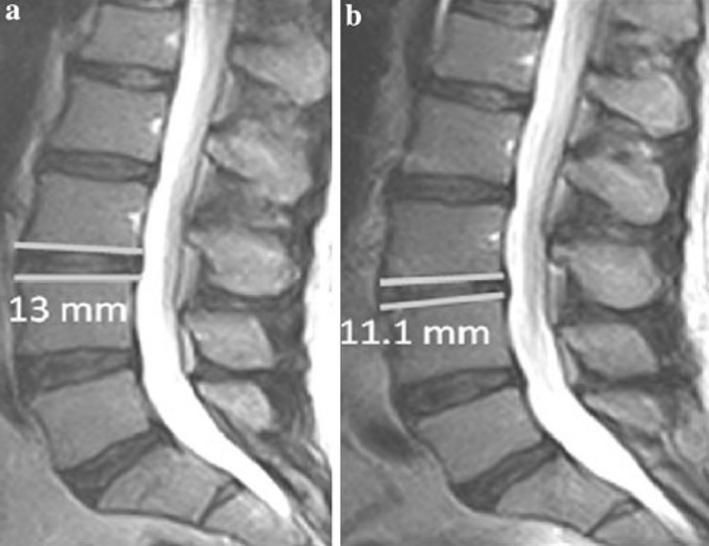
Fast spin echo (FSE) T2-weighted magnetic resonance images (MRI) in the sagittal plane. Assessment of changes in thickness of the intervertebral disc in the transition from a supine to b upright position, with averaged valuesInterspinous distance between L3 and L4 (Fig. 3a, b).
